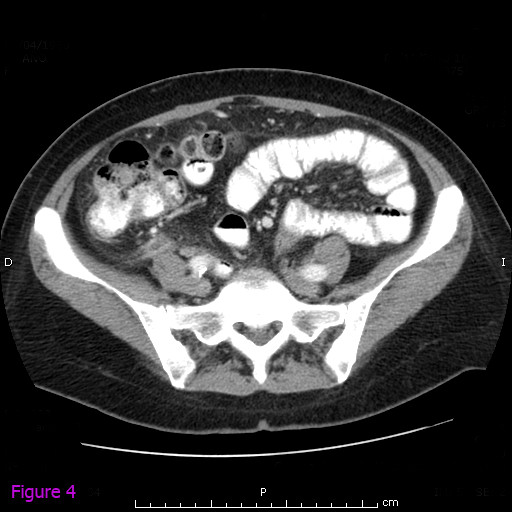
Contrast-enhanced computed tomographic scout view of the abdomen revealing the retrocecal region of the appendix with inflammation and mesenteric fat infiltration and a thickened appendiceal wall, suggesting acute appendicitis.
